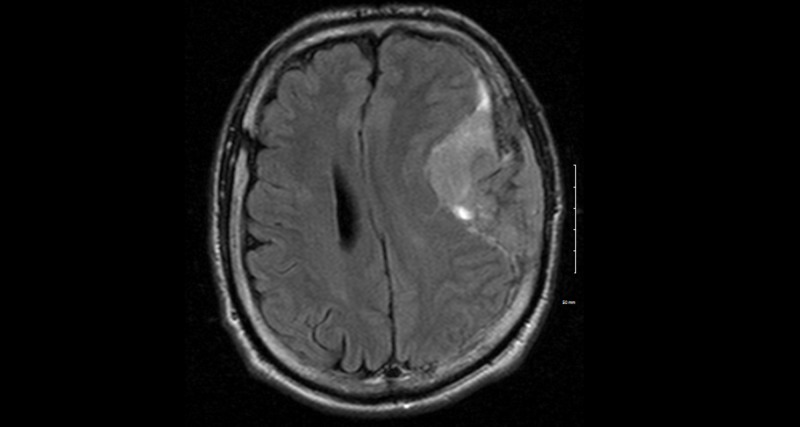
Axial T2 FLAIR post-contrast sequence showing large left frontal/parietal extra-axial enhancing lesion FLAIR: fluid-attenuated inversion recovery
